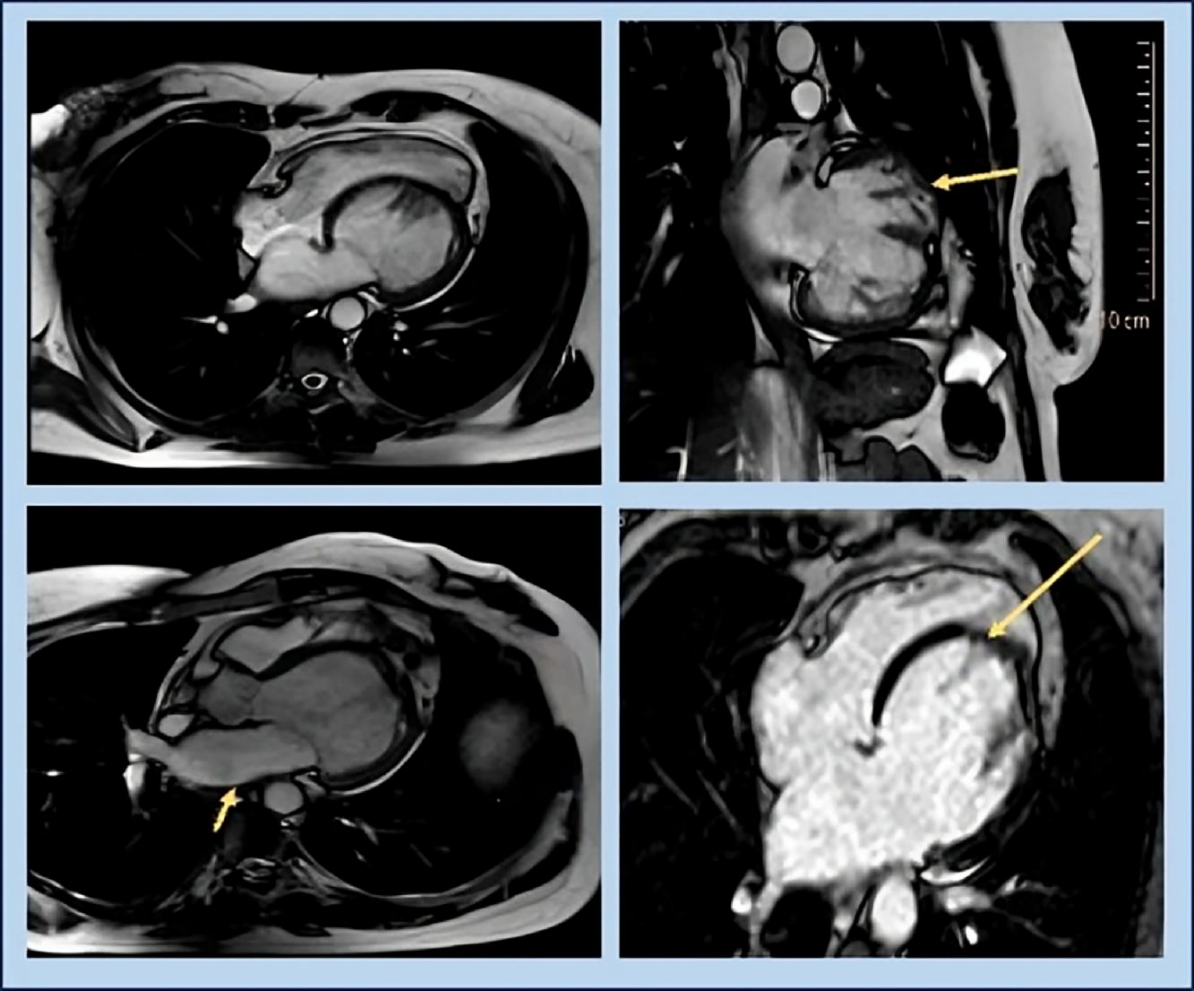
Cardiac MRI images suggestive of (*A*) cine 4 CH SSFP image that revealed globular and truncated left ventricle with elongated right ventricle which wraps around the deficient left ventricle apex and IVS bulging towards the right ventricle, (*B*) complex pattern of papillary muscles with bifid anterior and posterior papillary muscles with normal chordae attachment, (*C*) mildly dilated left atrium, and (*D*) delayed enhancement IR-GRE 4CH image showing no delayed myocardial hyperenhancement.
